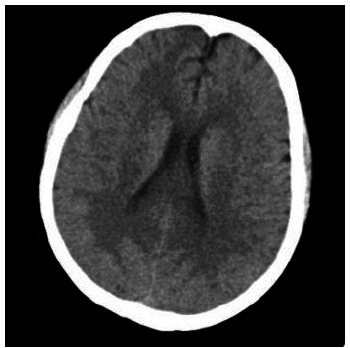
CT brain imaging on the day of onset Cranial CT examination suggested bilateral cerebral hemispheric symmetry, normal gray and white matter contrast, absence of abnormal density in the brain parenchyma, and no hemorrhage, infarction, or other new lesions.
